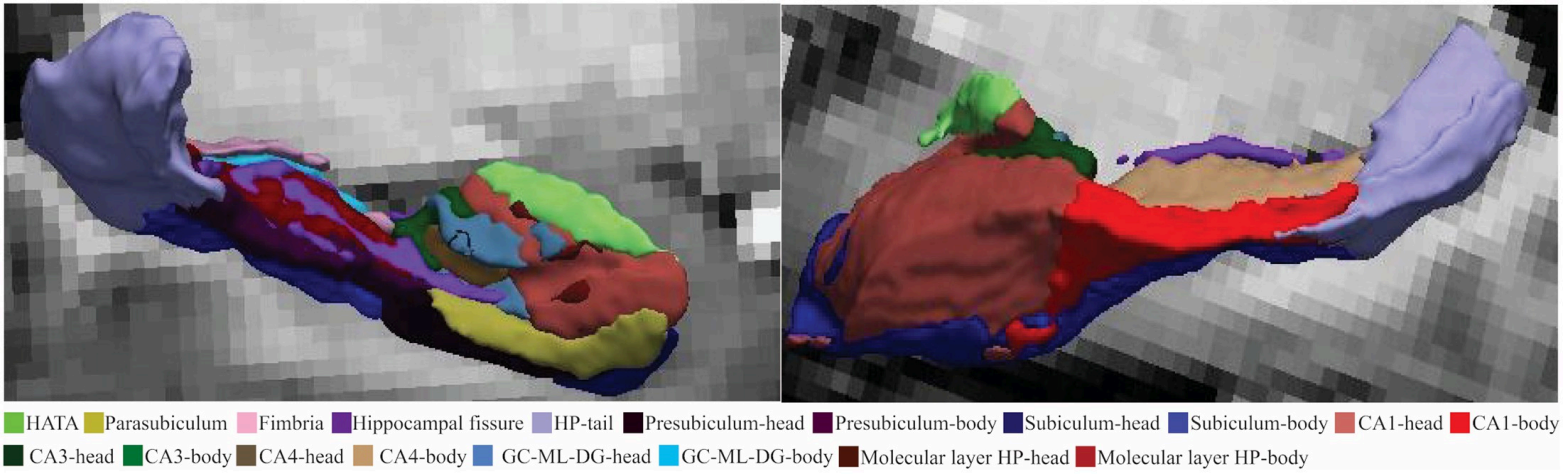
Hippocampal subfield segmentation of a healthy participant overlaid on a reference T1-weighted image. Different colors representdistinct subfields.
